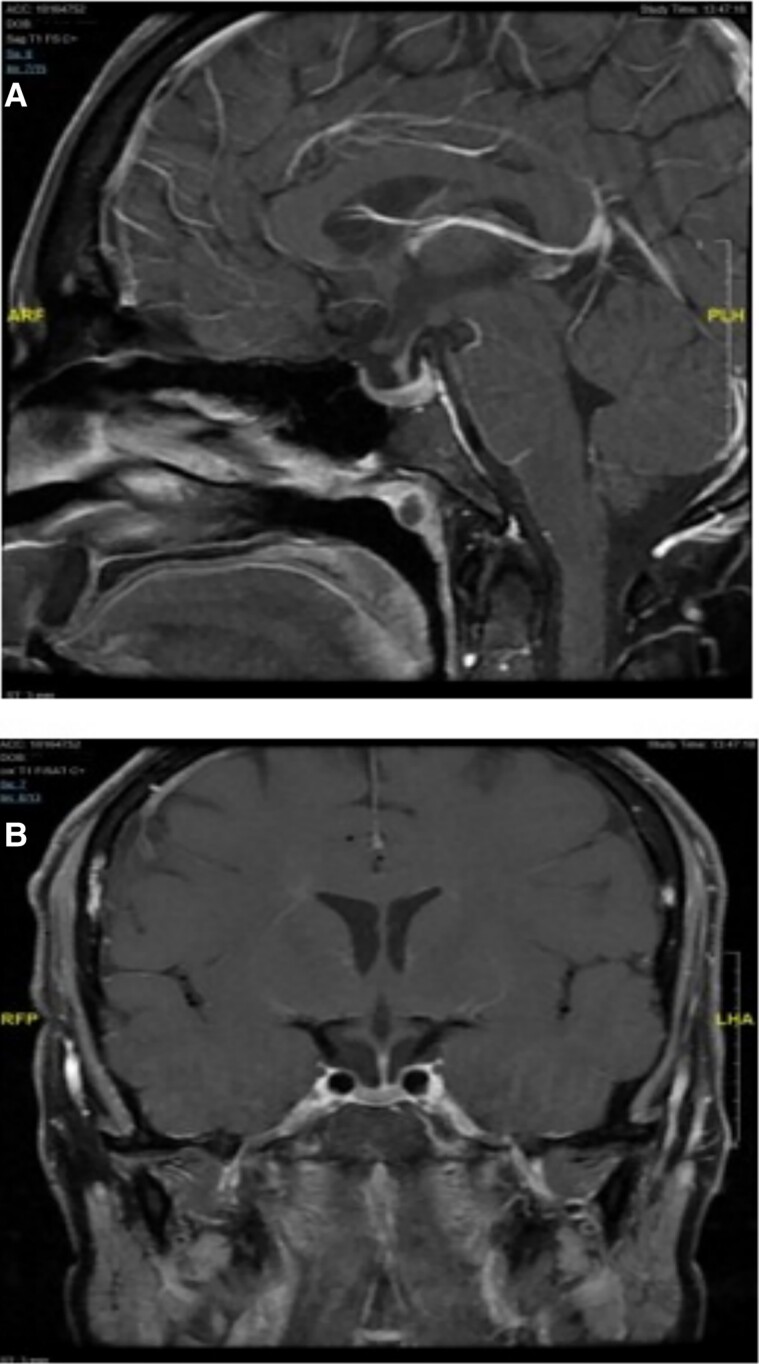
Gd-DTPA–enhanced pituitary magnetic resonance imaging scan 6 months after initiating thyroid hormone replacement therapy. (A) and (B) Sagittal and coronal images displaying a significant reduction in pituitary size.
